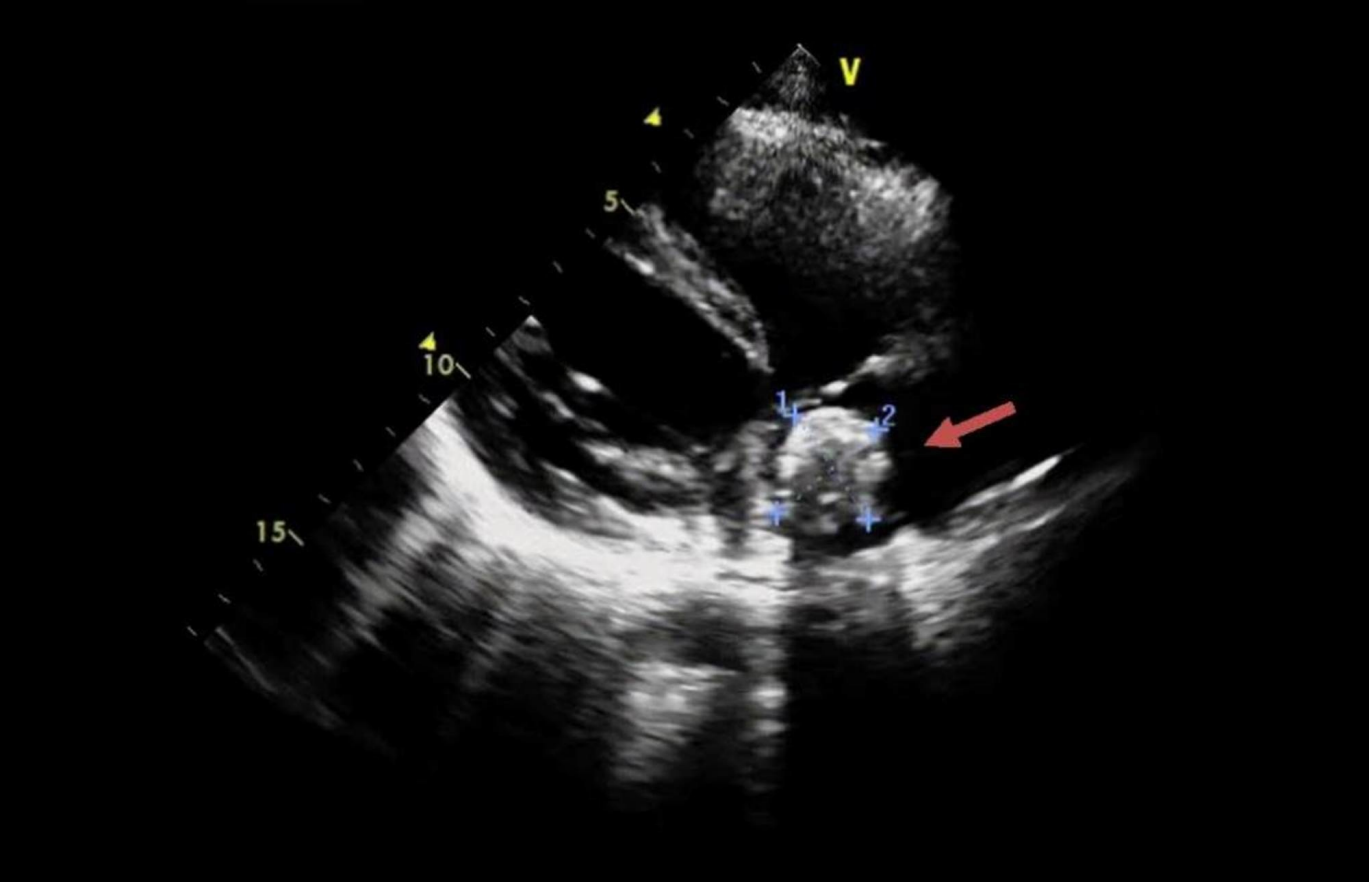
Two-dimensional transthoracic echocardiogram. Red arrow shows a well-defined 28 × 28 mm heterogeneous consistency attached to right side of the right atrium in fossa ovalis.
